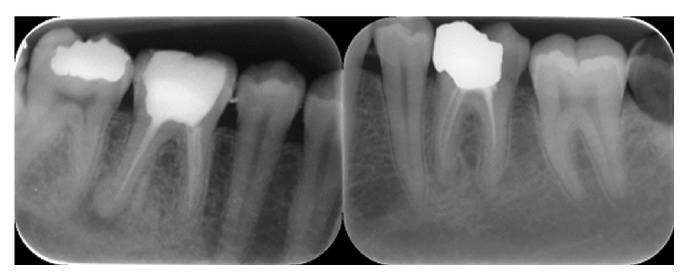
Teeth with gutta-percha more than 2 mm short of the radiographic apex (underfill).
